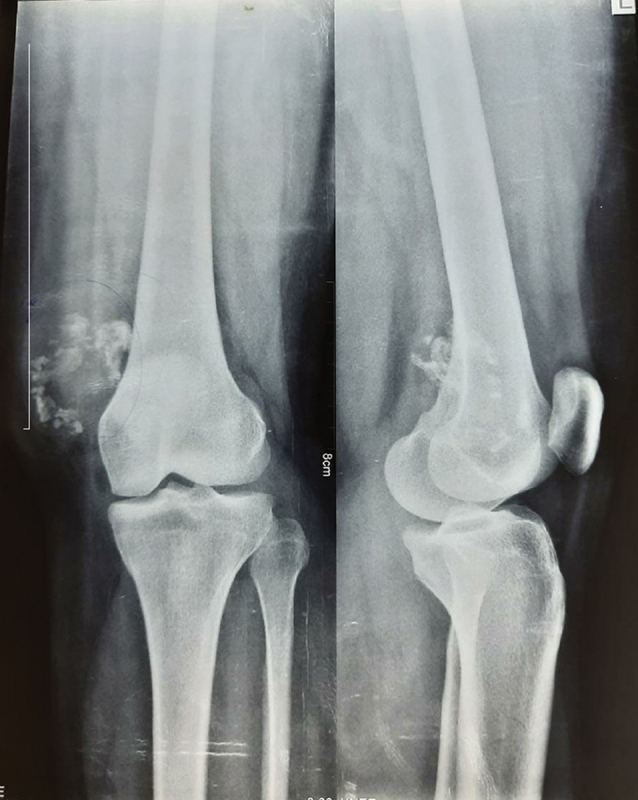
X-ray image of a 25-year-old female patient who presented with slow-growing swelling of the left knee. The radiograph showed a soft-tissue lesion in the anteromedial aspect, with multiple punctuate and circular calcification.
